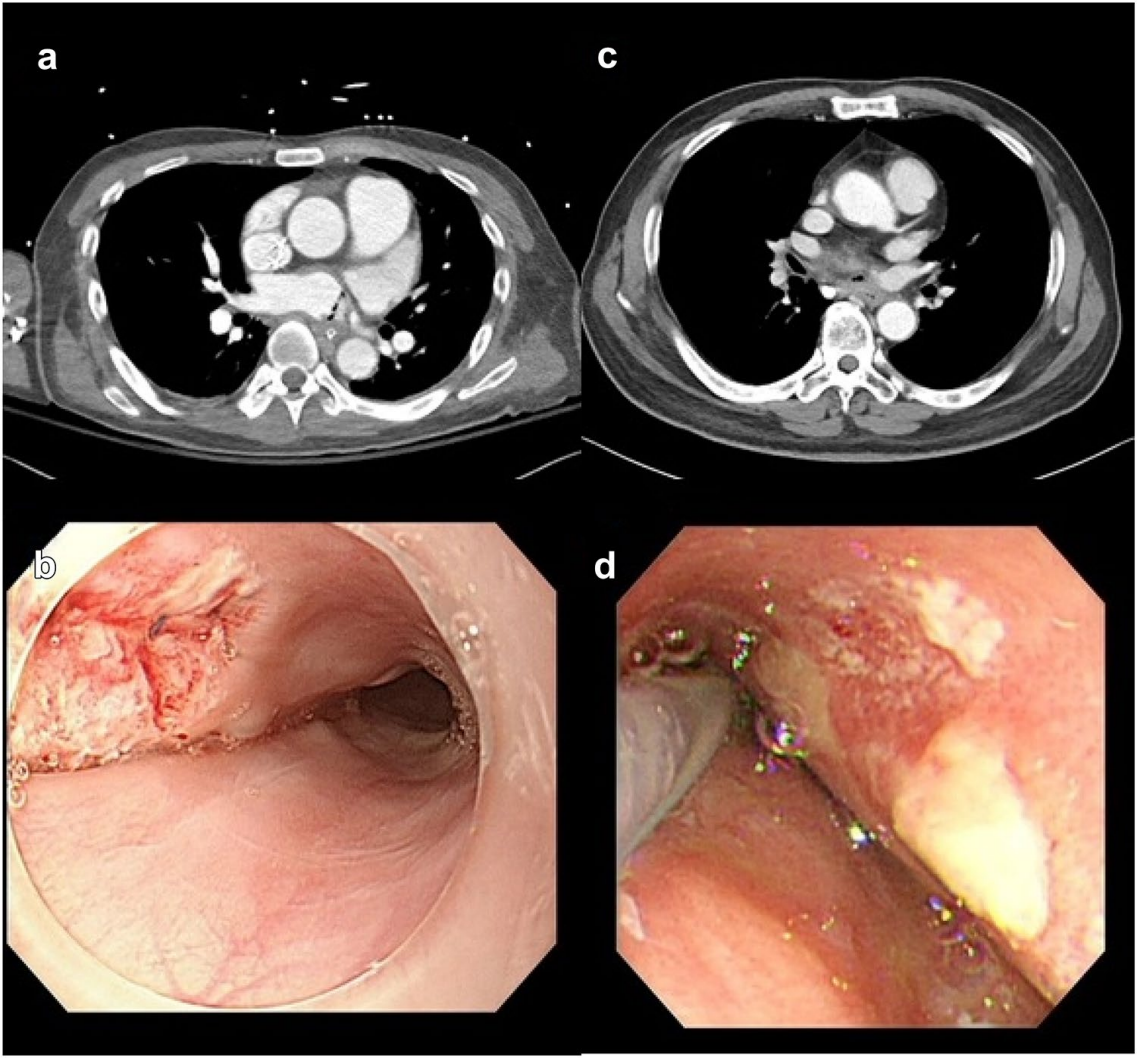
Perioperative findings about atrial-esophageal fistula in two patients. a A CT scan of the chest showing abnormal linear air densities in the left atrium demonstrating an AEF in case 1. b An endoscopic finding with a well-repaired previous fistula site in case 1. c A CT scan of the chest revealing air bubbles at the mediastinum suggestive of an AEF in case 2. d An endoscopic finding was unremarkable for a prior AEF site covered with a whitish exudate scar in case 2
